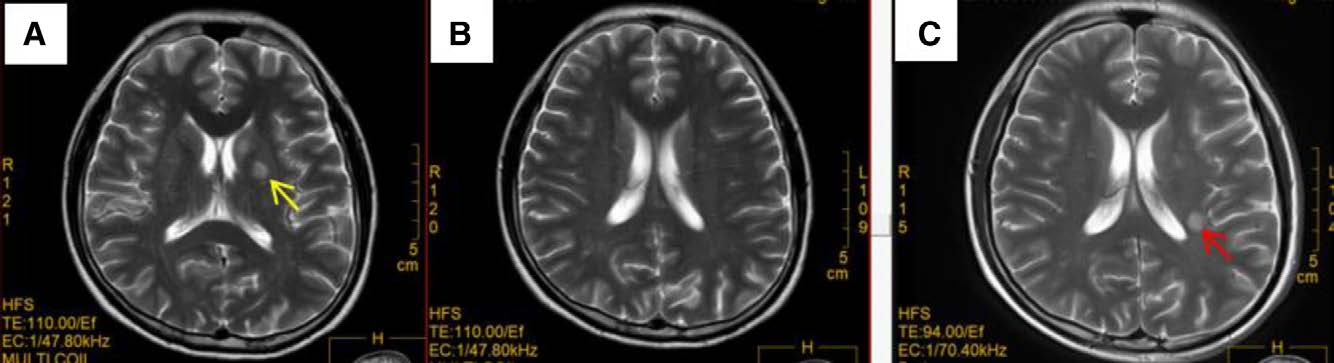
Infection-related brain MRI. (A and B) Brain MRI scan on April 30, 2024: showing abnormal signals (yellow arrows). (C) Follow-up brain MRI scan on May 30, 2024, revealed new lesions (red arrow). MRI = magnetic resonance imaging.
